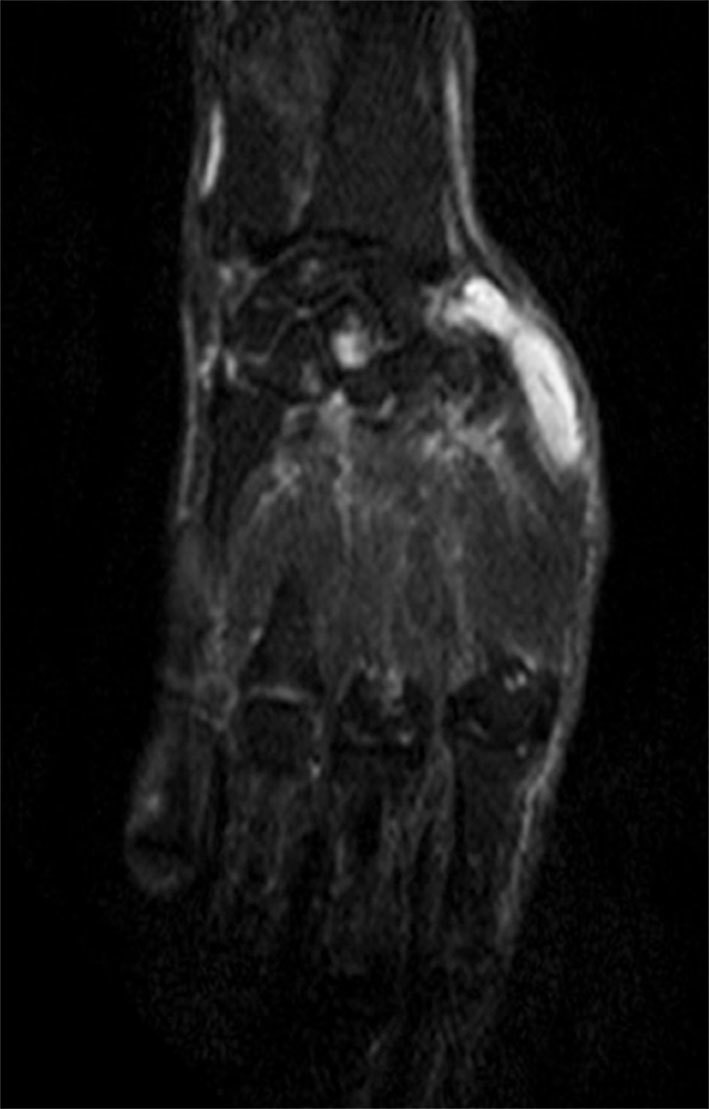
Coronal short tau inversion-recovery MRI of the right hand showing a hyperintense cystic lesion within the capitate and the lunate.
